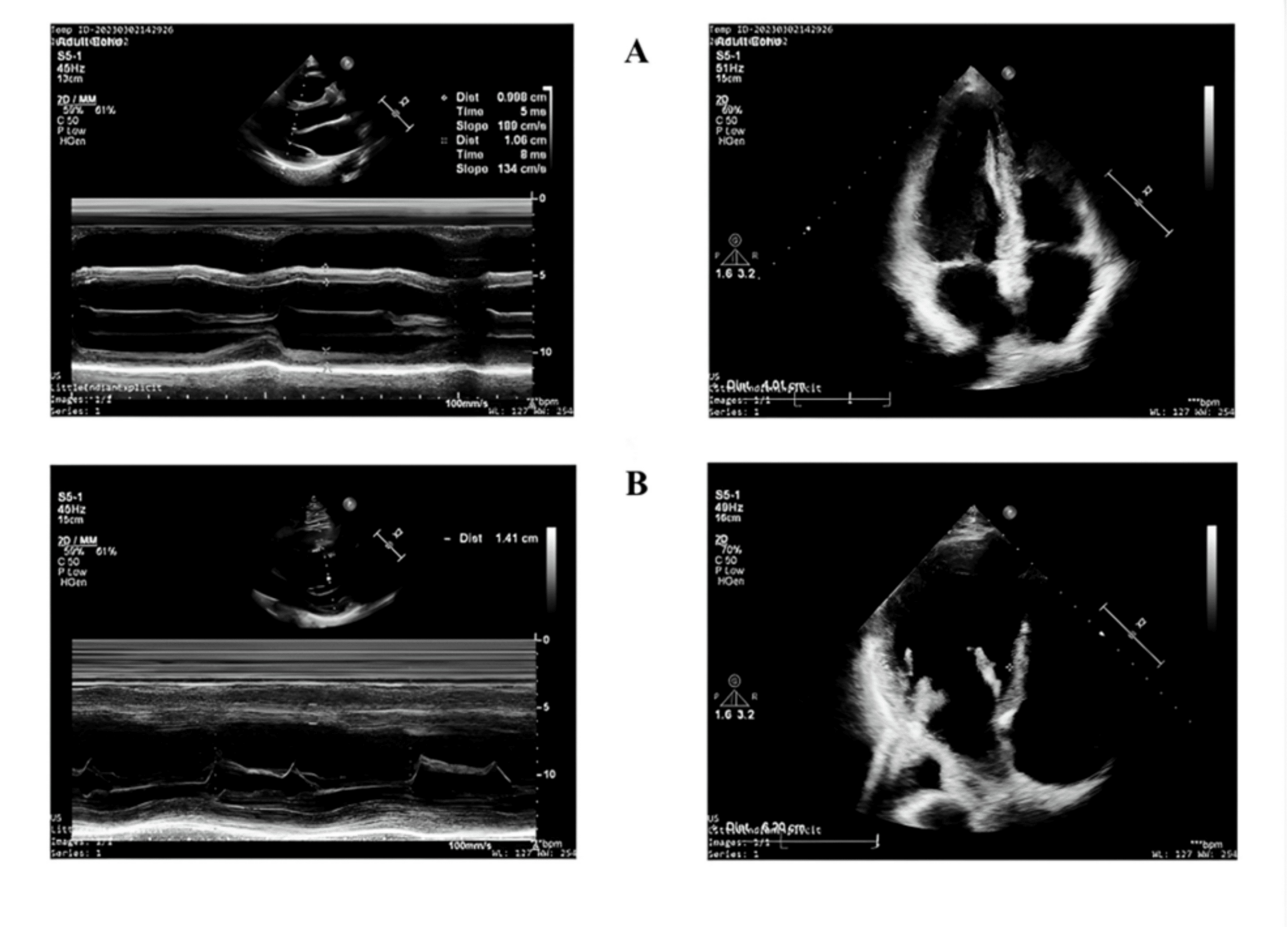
Hypertrophic changes associated with aminothiol dysregulation (A) Two-dimensional transthoracic echocardiographic image of a CABG patient with tHcy < 12 µM/L. Left panel: parasternal long axis view: normal thickness of IVS and posterior wall can be seen (10 mm). Right panel: Apical four-chamber view shows a normal LVED (40 mm). (B) Two-dimensional transthoracic echocardiographic image of a CABG patient with tHcy > 12 µM/L. Left panel: Parasternal long axis view: considerably increased (14 mm) thickness of IVS and posterior wall can be seen. Right panel: Apical four-chamber view shows an increased LVED (60 mm). LVED: left-ventricular end-diastolic diameter; CABG: coronary artery bypass grafting; tHcy: total homocysteine (reduced + oxidized); IVS: interventricular septum Image Credit: Cziraki et al., 2023 [23]; published under CC BY 4.0, Attribution 4.0 International Deed (https://creativecommons.org/licenses/by/4.0/)
